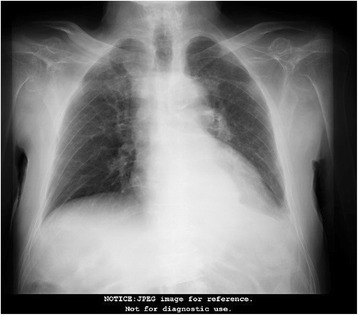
Chest X-ray on admission. Pleural fluid in the left basal lung area and dorsal vertebral column with signs of osteopenia
